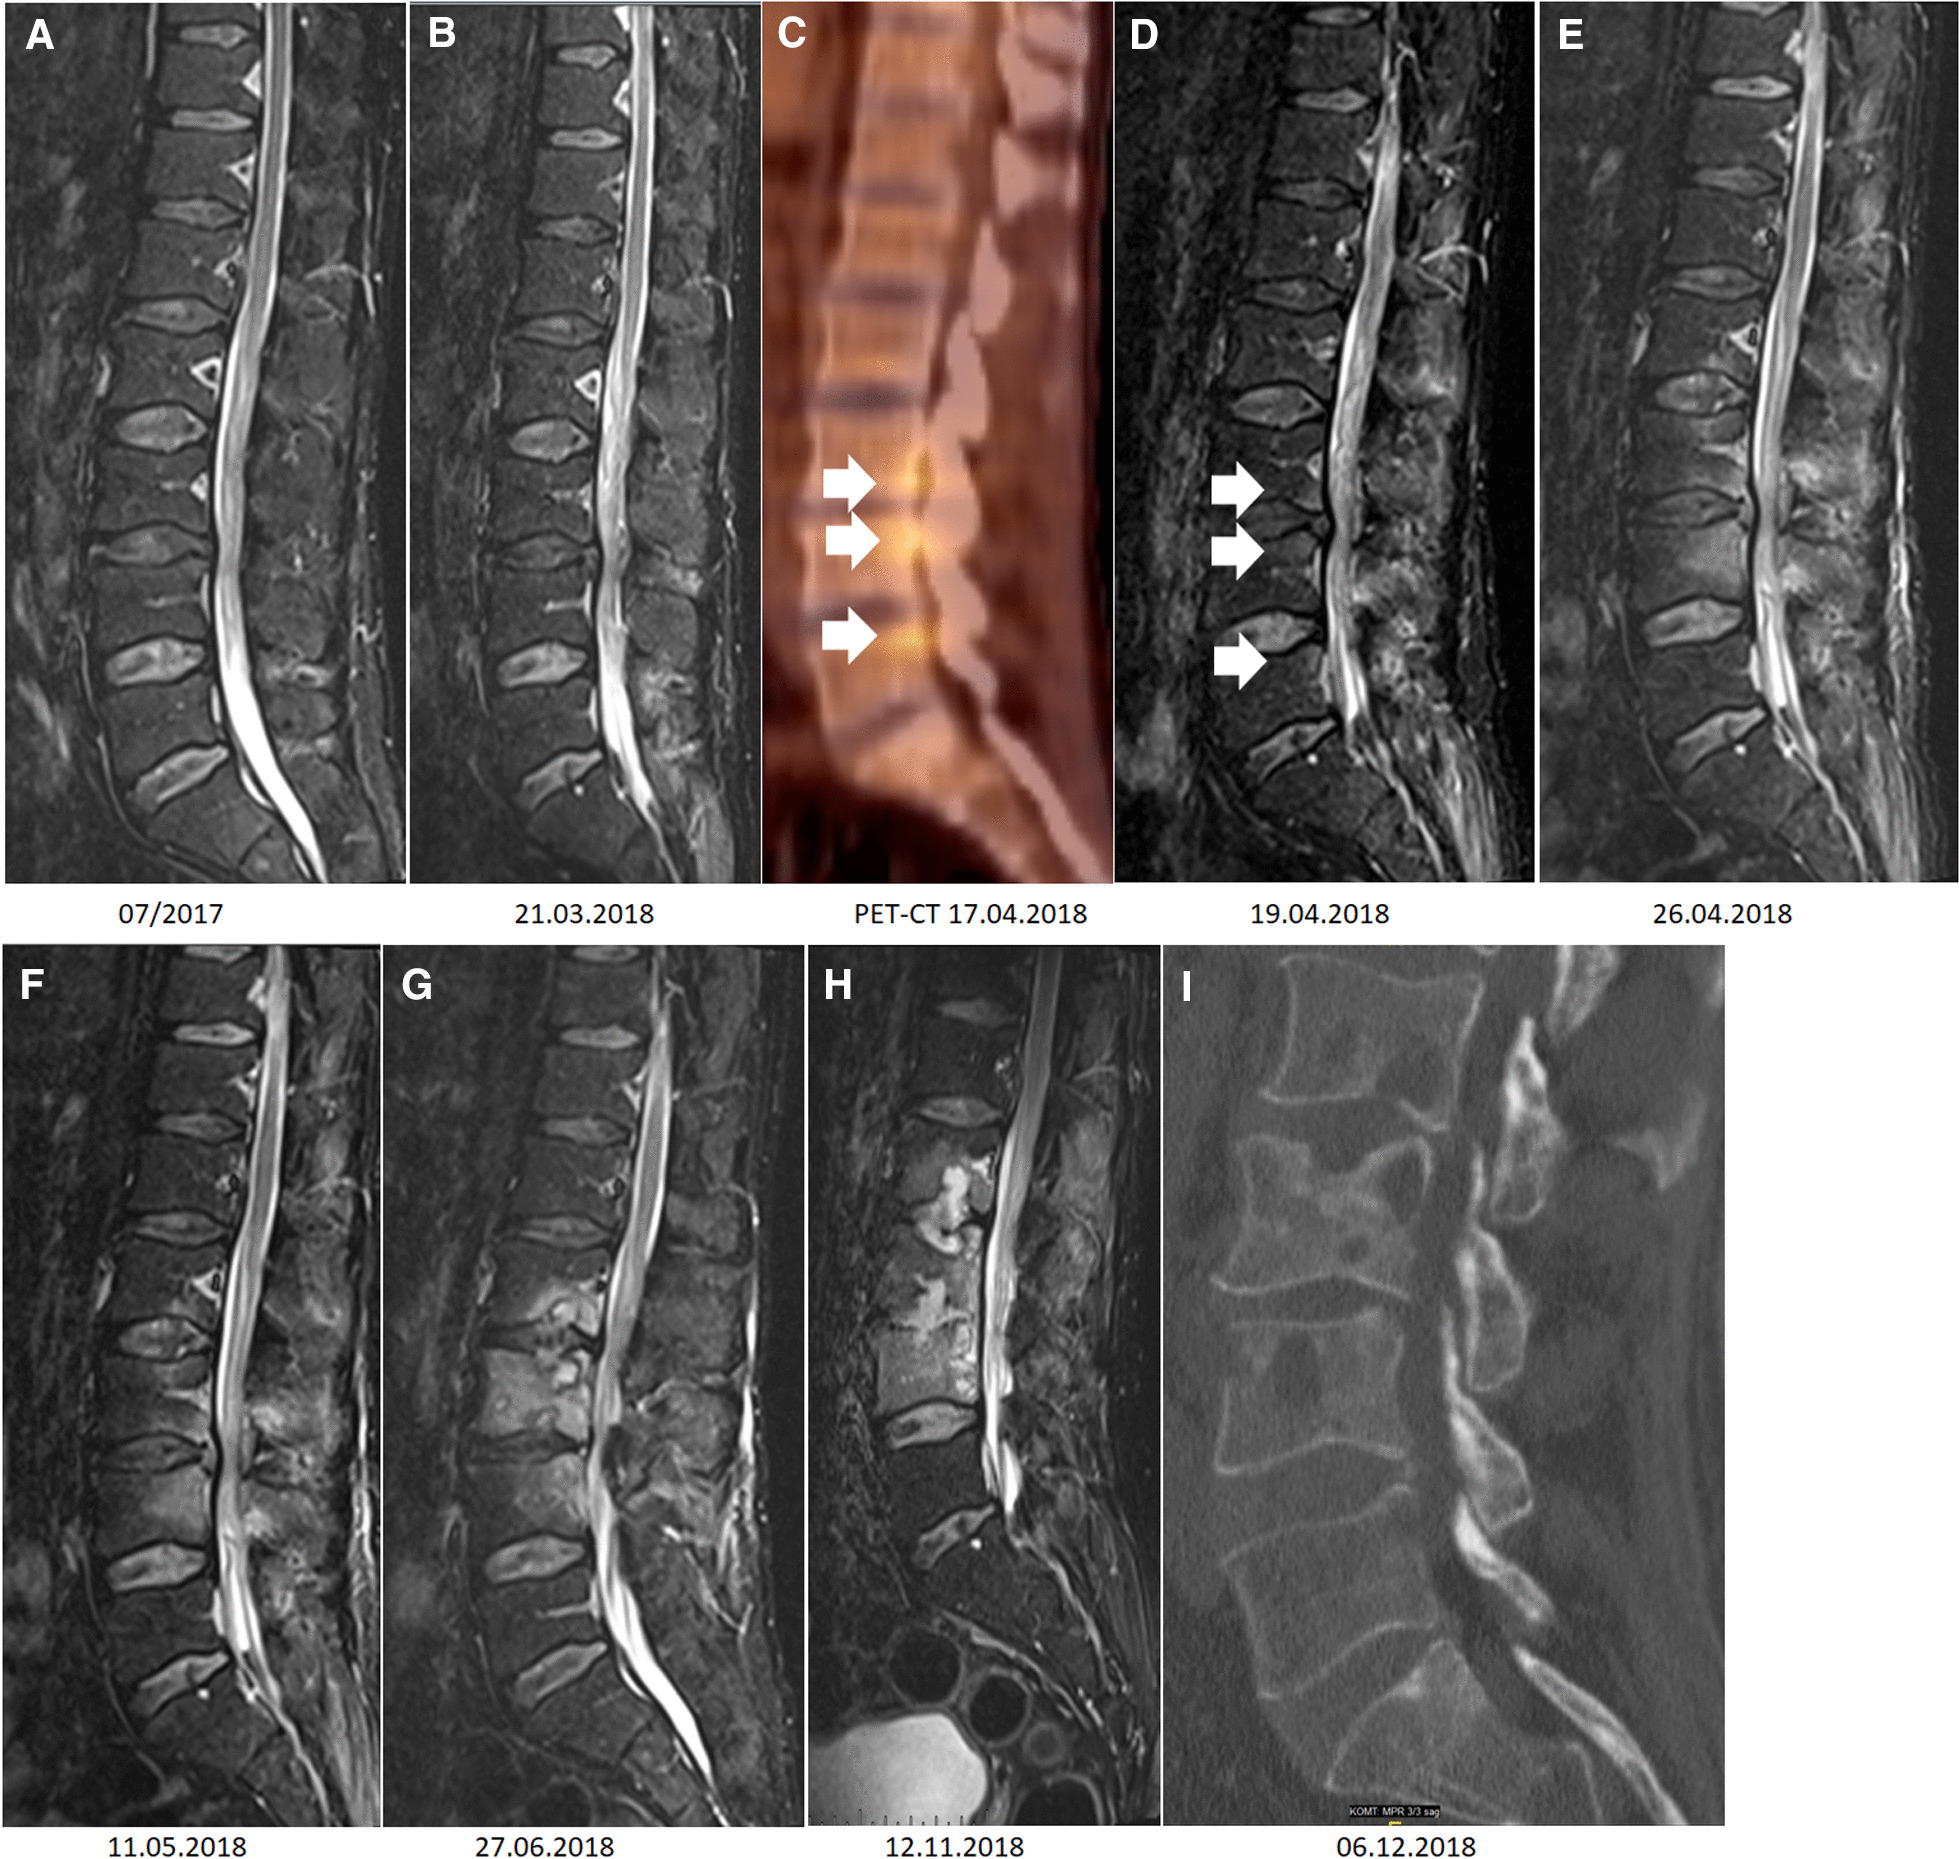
a–i MRI over the course and PET/CT. a Unremarkable sagittal STIR sequence of the lumbar spine from July 2017 with former radicular symptoms. b Multisegmental degenerative changes to the lumbar spine with progressive disc herniation at the level of L3/4. c Fluorine-18-fluorodeoxyglucose positron emission tomography (18F-FDG PET/CT) with hot spots with increased glucose activity dorsally on inferior endplate L3 and dorsally on superior endplates L4 and 5 (short white arrows). d Sagittal STIR sequence of the lumbar spine. Oedema of the endplates consistent with the PET/CT from c (short white arrows). e–h. Sagittal STIR sequence of the lumbar spine. Progressive oedema of L2-4 and regression of oedema in L5. Increasing destruction of the endplates within the context of spondylodiscitis. i Sagittal reformatted CT showing partial sclerosis of the vertebral bodies L2–L4. Furthermore, sharply demarcated lytic areas mainly associated with the endplates
